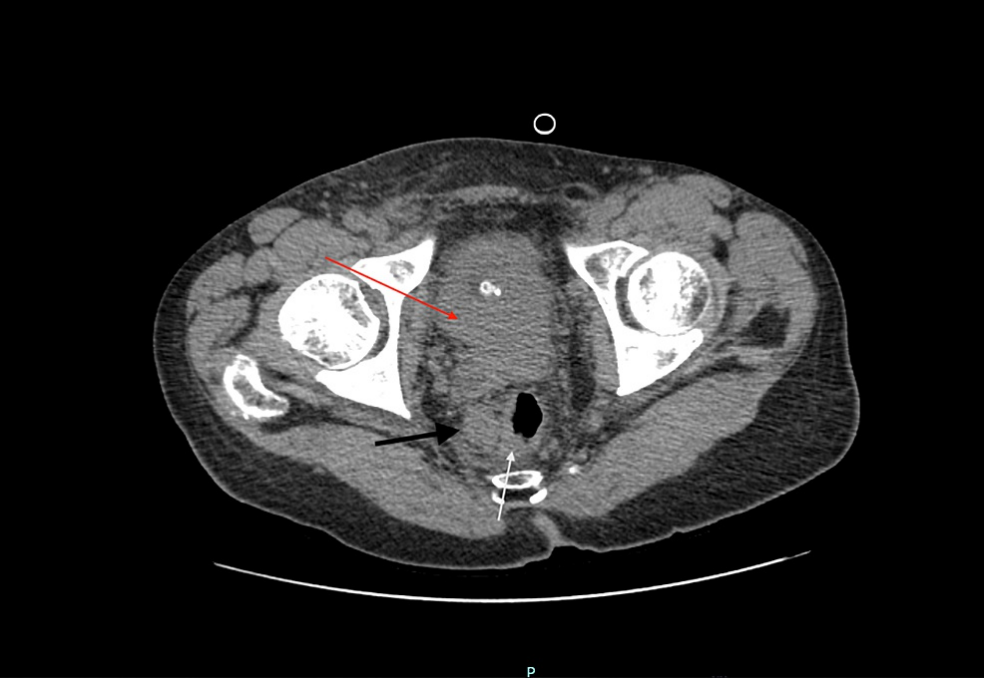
CT scan of the abdomen White arrow shows enlarged and irregular prostate; Black arrow shows prominent perirectal lymph node; Red arrow shows bladder wall thickening
